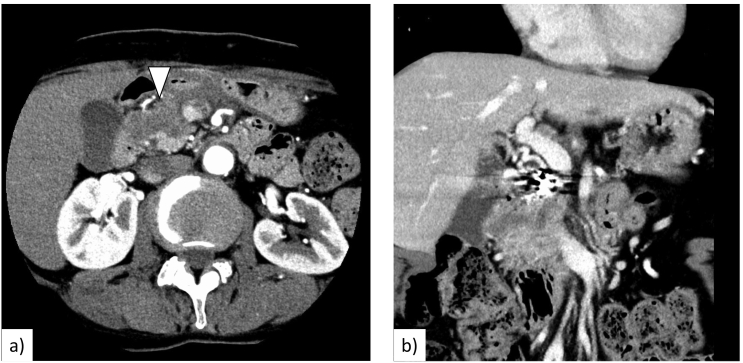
a. A CT scan after chemotherapy revealed that the pancreatic mass had shrunk to 29 mm (arrowhead). b. The border between the tumour and portal vein was unclear.
